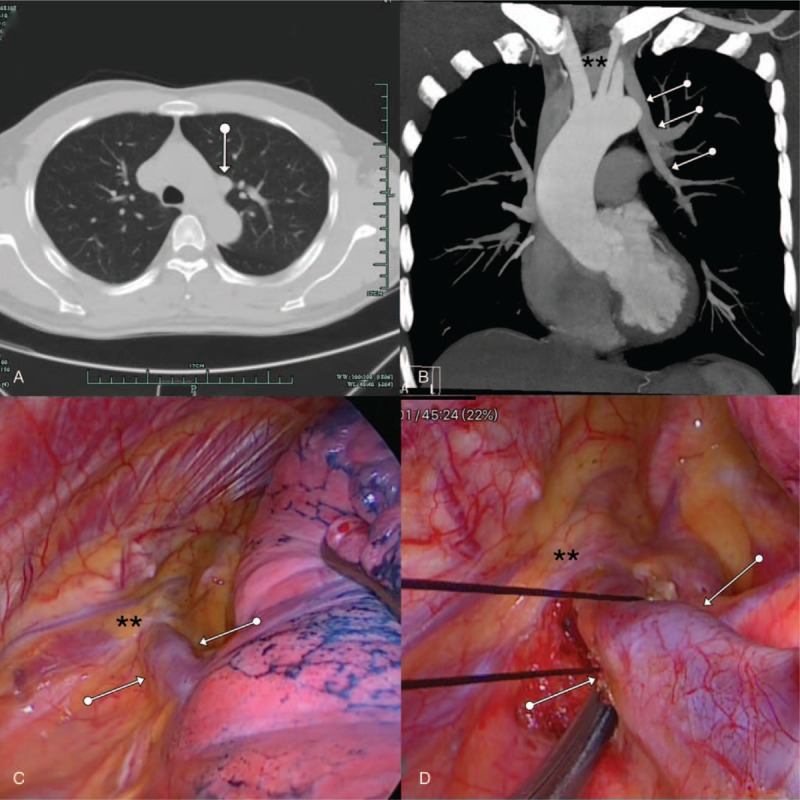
(A) An anomalous vessel were found near the aortic arch while no malignant tumor, tuberculosis, pneumonia, or bronchiectasis were detected by chest CT; (B) Contrast-enhanced chest CT revealed that the left upper pulmonary vein (white arrows) drained into the left brachiocephalic vein (asterisks); (C, D) Intraoperatively, the left upper pulmonary vein (white arrows) was confirmed draining into the left brachiocephalic vein (asterisks). CT = computed tomography.
